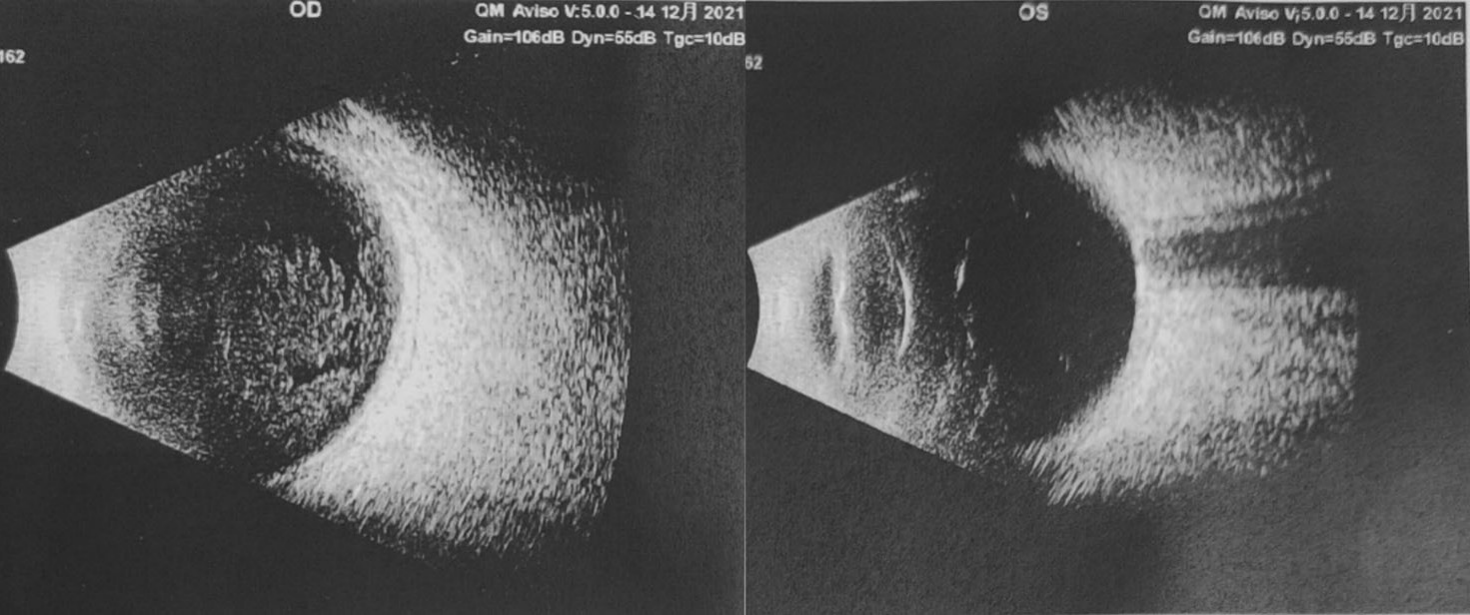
B‐scan ultrasonography revealing vitreous opacity and vitreous infiltration at the posterior hyaloid membrane, no retinal detachment in the right eye.
